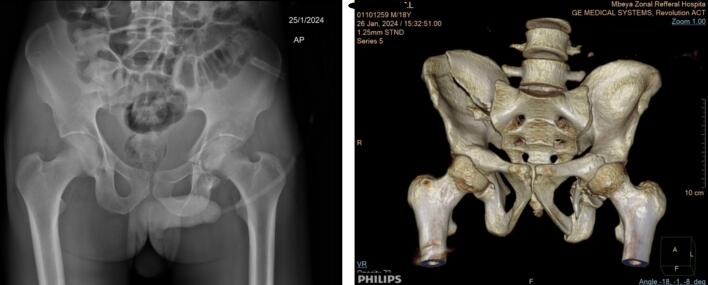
Left; A plain radiograph of the patient showing a right iliac wing fracture, which is not clearly visible due to bowel overshadowing and a right acetabular anterior and posterior column fracture. Right; A 3D reconstruction image revealing a comminuted displaced fracture of the right iliac bone extending to the sacroiliac joint (SIJ) articular surface, along with left acetabular anterior and posterior column fractures and a quadrilateral plate fracture.
